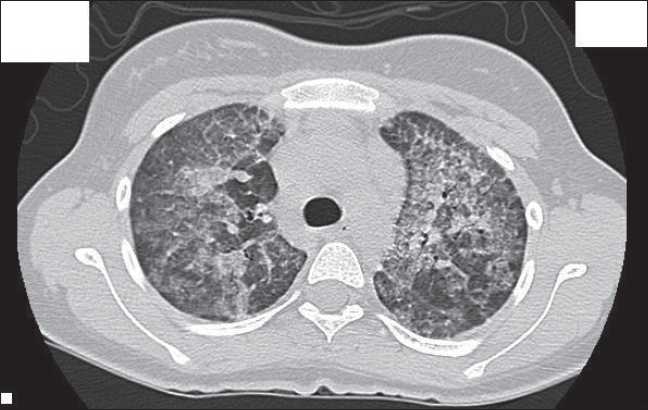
CT lung scan showing crazy paving pattern
